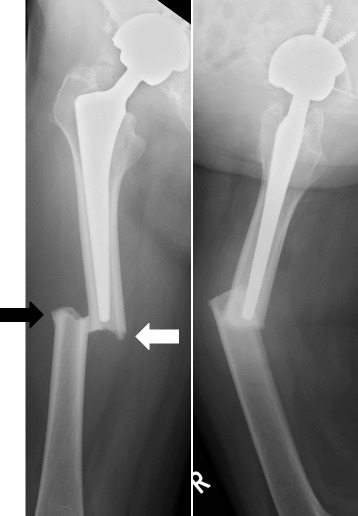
Radiographs demonstrate a periprosthetic femoral fracture with major radiographic features of an atypical femoral fracture. Note the localized periosteal thickening of the lateral cortex (black arrow) and the medial spike (white arrow).
